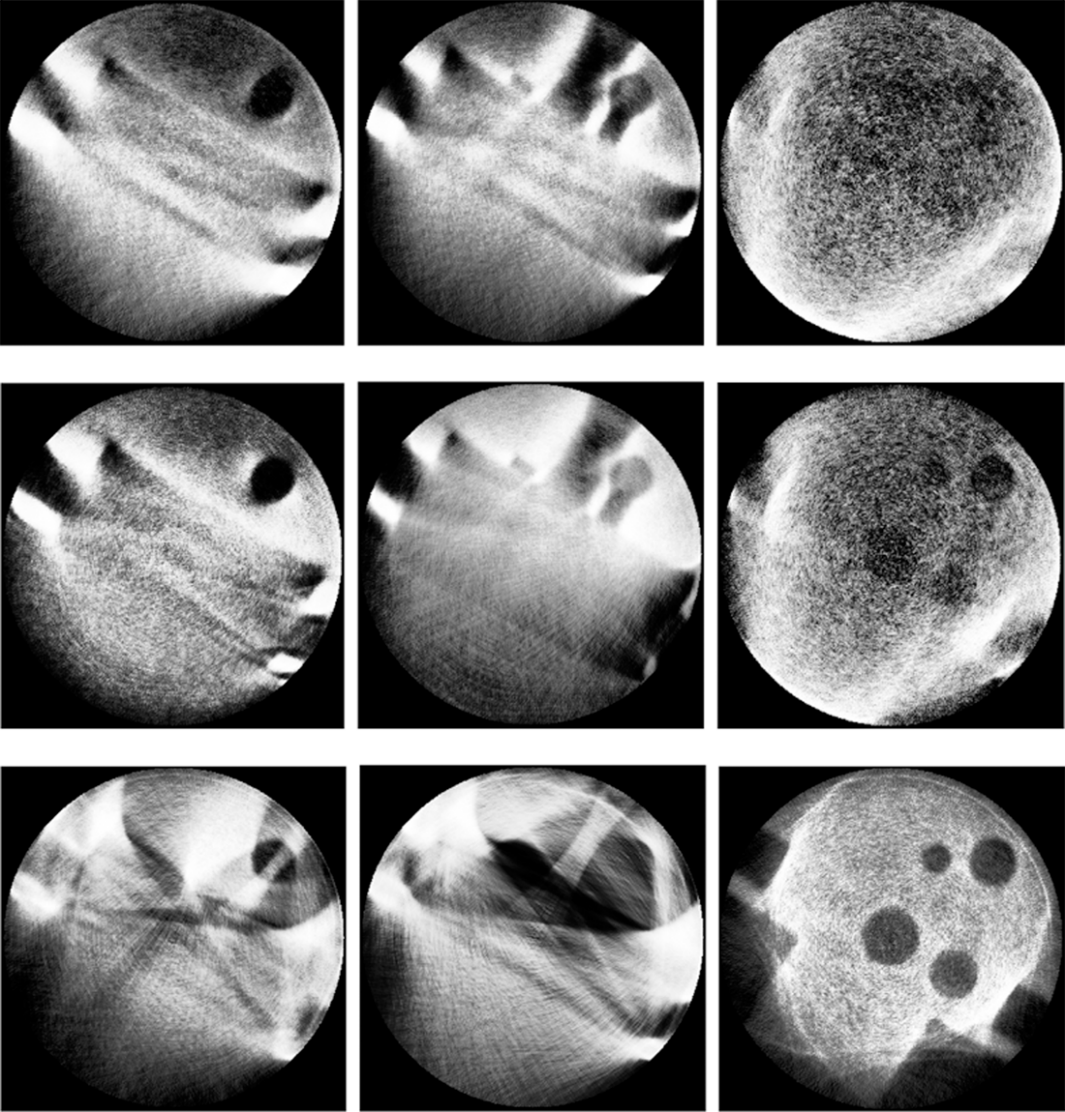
Single-voltage CT images with different energy and different part. Every column is the 60kV, 80kV and 100kV at the same prat. Every row is the bottom, center, and top part at the same voltage.
